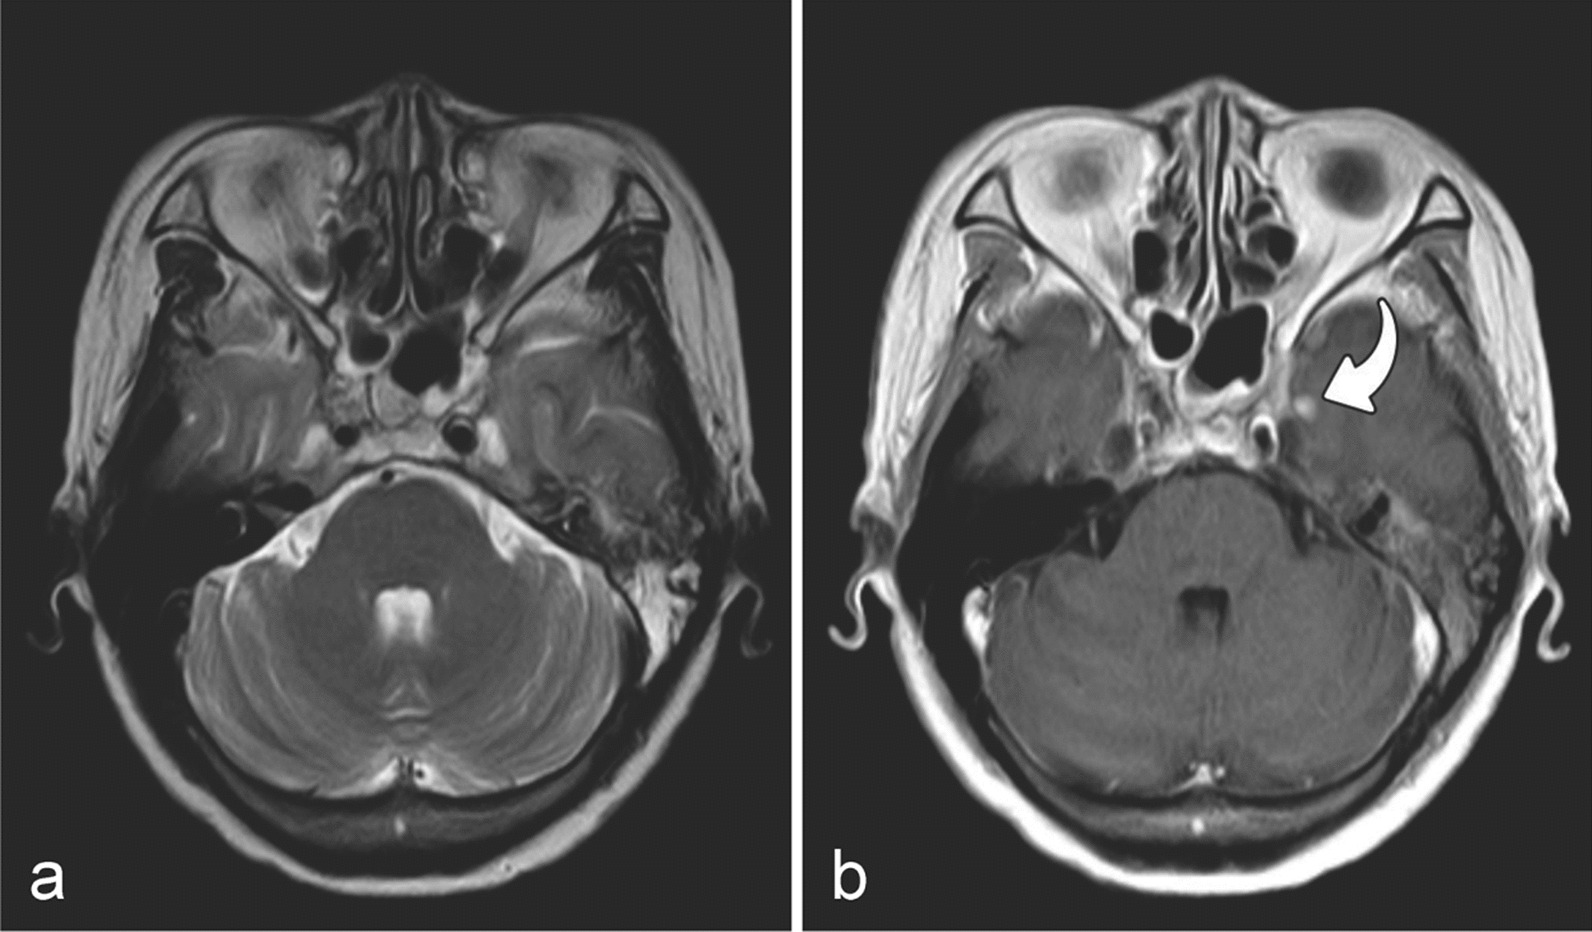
MRIs of a patient with RTLI (46y, M). MRIs of a patient with RTLI (46y, M) with the earliest MRI finding of solid enhanced nodule at 10.87 months after receiving IMRT. Axial T2-weighted image shows slightly high intensity in left temporal lobe (a), and a solid enhanced nodule without cystic necrosis is seen in the inferomedial part of the left temporal lobe on the axial post-contrast T1-weighted image (b) (curved arrow). *Note*: *MRI* magnetic resonance imaging, *RTLI* radiotherapy-induced temporal lobe injury, *IMRT* intensity-modulated radiotherapy
